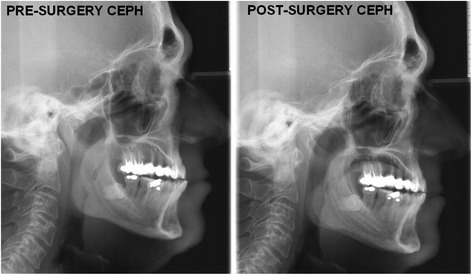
Comparison of lateral cephalometric x-ray previous to tumor resection and 12 months later. Note the normalization of the morphology of the sella turcica and the improvement in the inclination of the maxillary incisors
